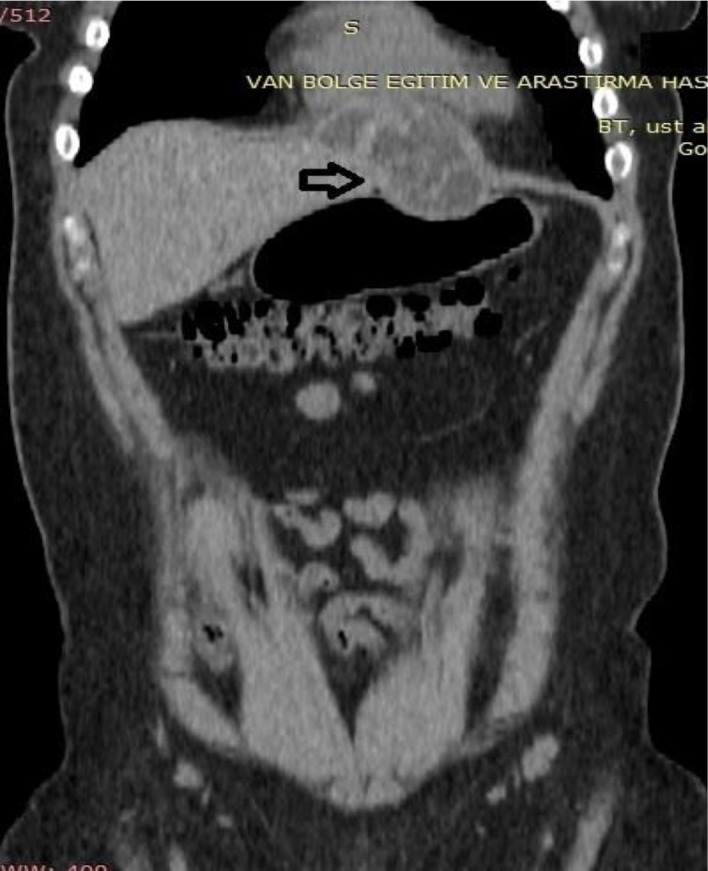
A coronal reformatted CT image at first admission shows type III hydatid cyst in left hepatic lobe which causes elevation of diaphragm and cardiac indentation.(Arrow)
